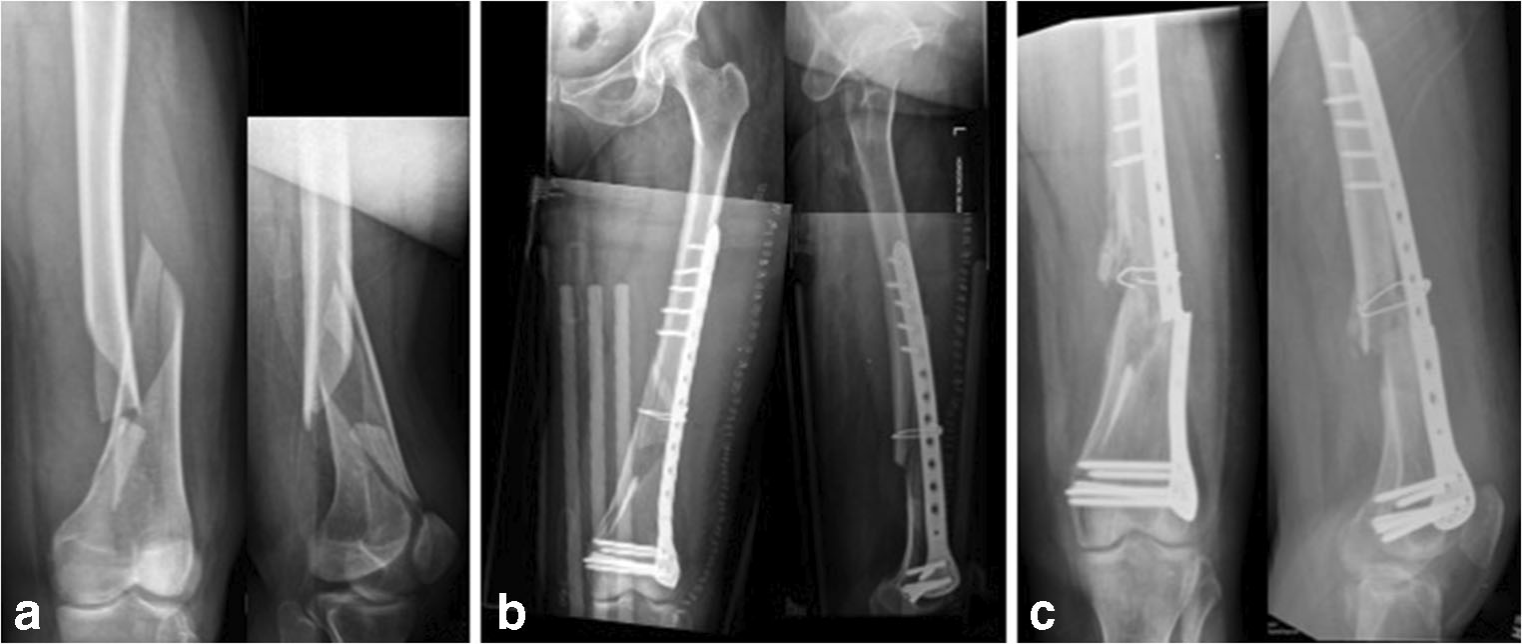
Patient 2: a Preoperative x-rays of distal third femoral diaphyseal fracture. b Post-operative x-rays with a locking plate with screws and a cerclage wire. c 9-month follow-up x-rays showing distal femoral non-union with implant breakage. The score calculated was 5 points (see Table 8)
